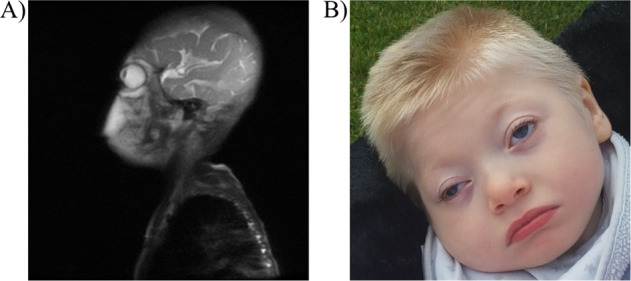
Clinical manifestations in patient V:7. A Magnetic resonance imaging showing lissencephaly with very thick cortex and dorsal dominance. As well as, some hypotrophy of vermis, while the frontal part of corpus callosum is well developed and the back part is thinner. B Patient V:7 showing dysmorphic features include bitemporal narrowing, wide mouth, puffy eyelids, broad mouth, high palate, two hemangiomas, microcephaly, short stature and overweight. For more detailed clinical description and phenotype comparison to other patients with Gustavson syndrome, see Supplementary data 1 and Supplementary Table 4B.
